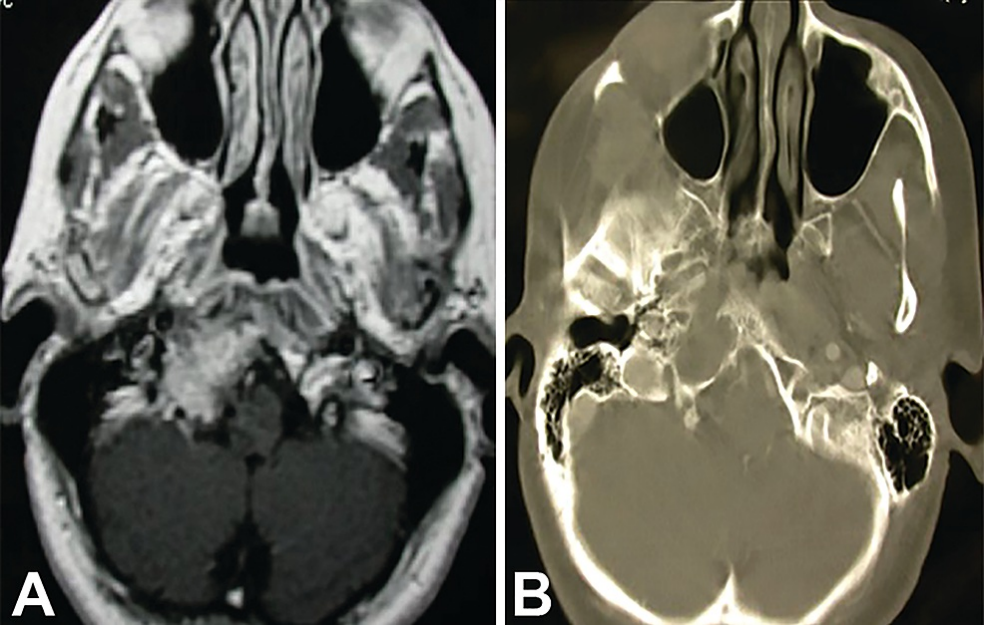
Plasmacytoma accessed via infra-hypoglossal triangle. (A) A contrasted T1 axial MRI and (B) axial CT scan demonstrating a lesion in the right occipitocervical joint. The patient complained of severe right-sided neck pain, and a modified approach was done exposing the lesion in the infra-hypoglossal triangle. Pathology was consistent with plasmacytoma (case 16).
